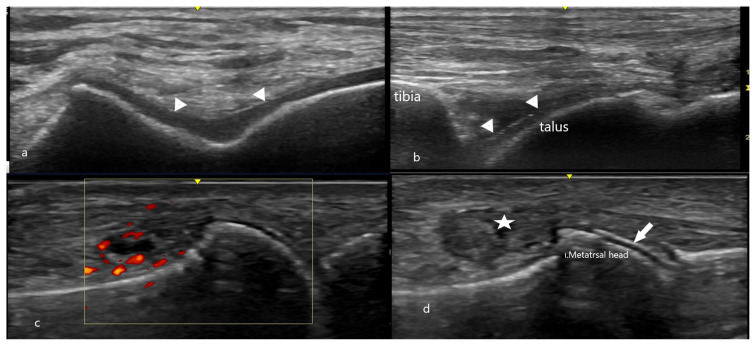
MSU crystals deposited on the cartilage surface: distal femur cartilage (a), talus cartilage (b), active gout arthritis, positive Doppler signal (c), and double contours seen in the 1st MTP joint in a gout patient (d). Arrowheads indicate double contours, and asterisks indicate a snowstorm.
